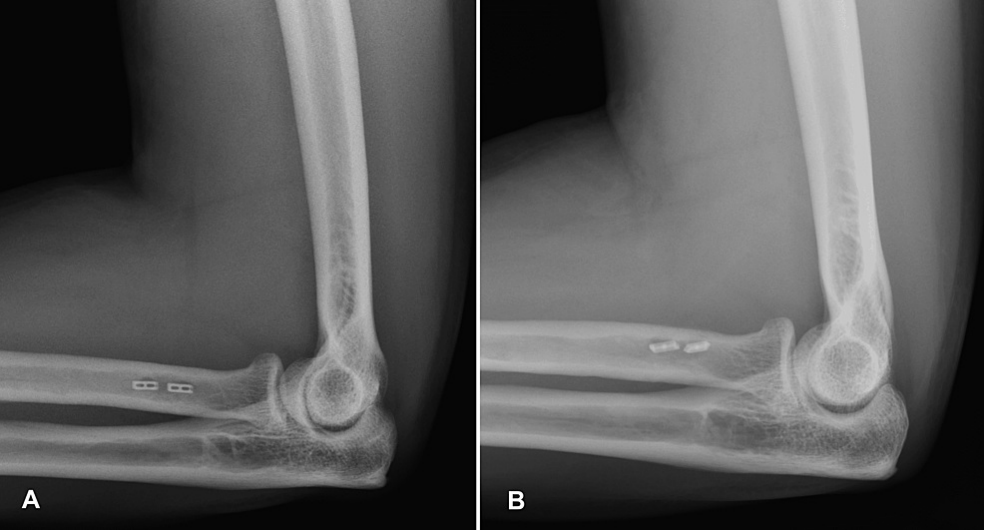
Postoperative lateral radiographs of the right (A) and left (B) elbows at the six-week follow-up showing stable hardware position
